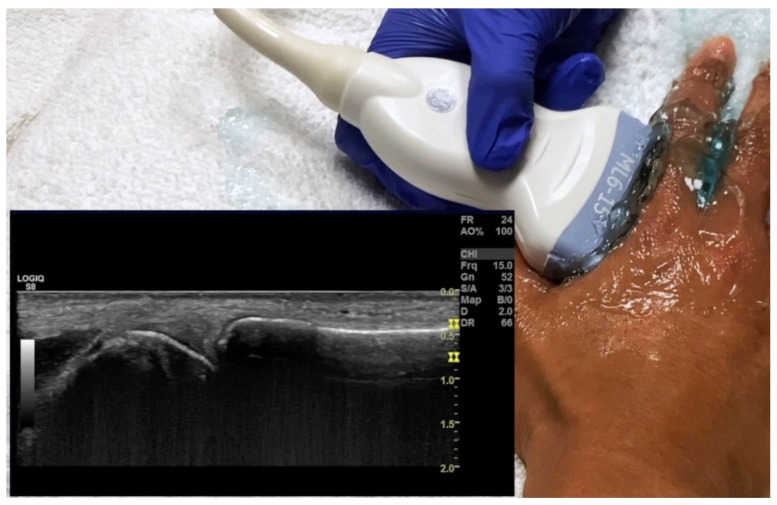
Probe placement and ultrasound of radial view of second MCP joint. MCP = metacarpal phalangeal.
